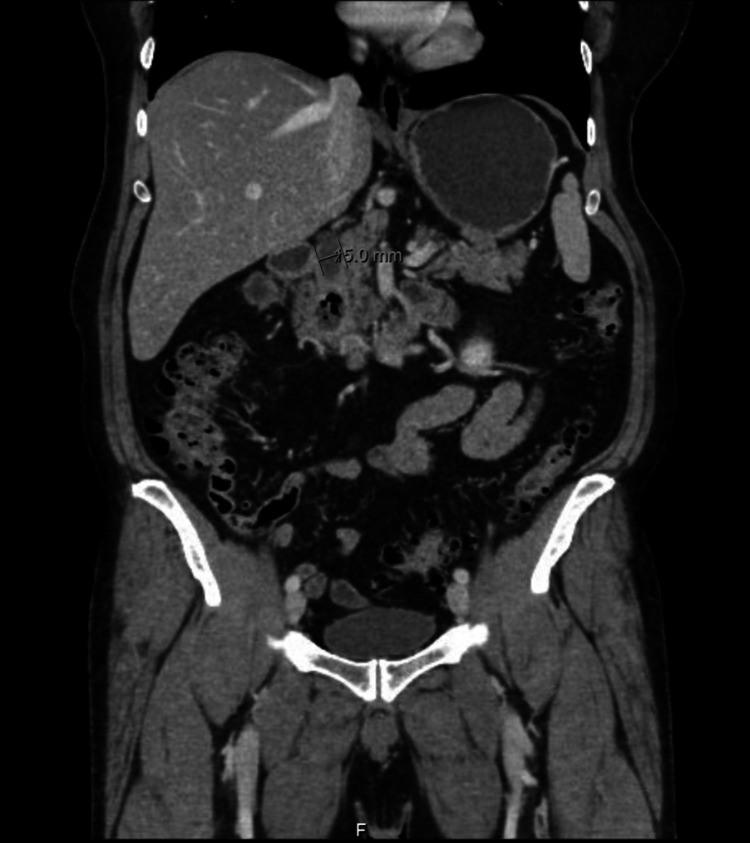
CT abdomen and pelvis (coronal view) showing a duodenal diverticulum measuring 5 mm and arising from the second part of the duodenum.
